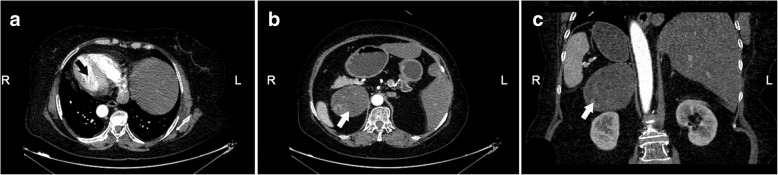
The CT findings. Situs inversus totalis was confirmed, and a mass of about 10 cm in diameter in contact with the right adrenal gland was detected. a Dextrocardia (black arrow: cardiac apex). b The axial plane (white arrow: the targeted adrenal mass). c The coronal plane (white arrow: the targeted adrenal mass)
